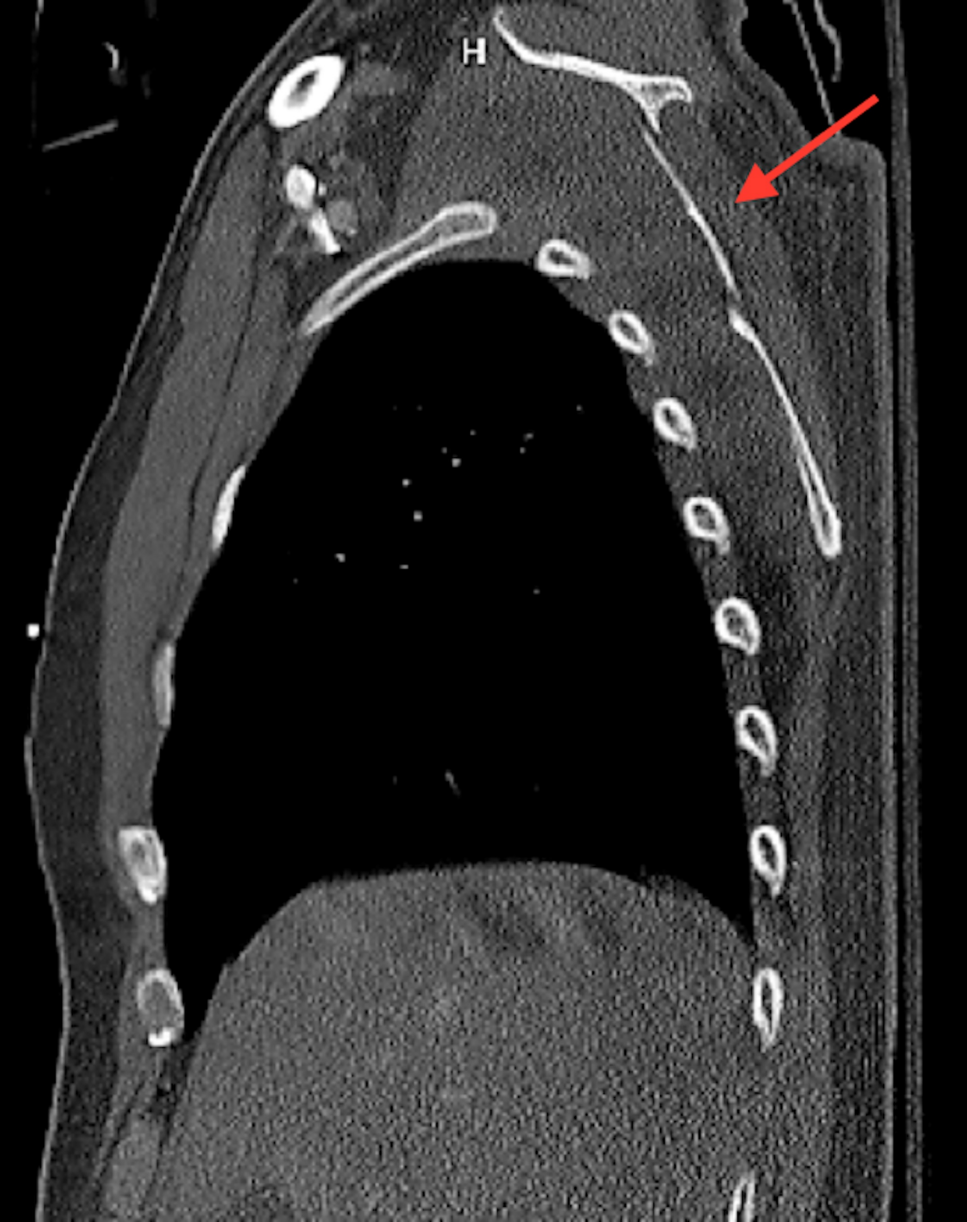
Sagittal CT demonstrating a right comminuted scapular fracture CT: computed tomography
